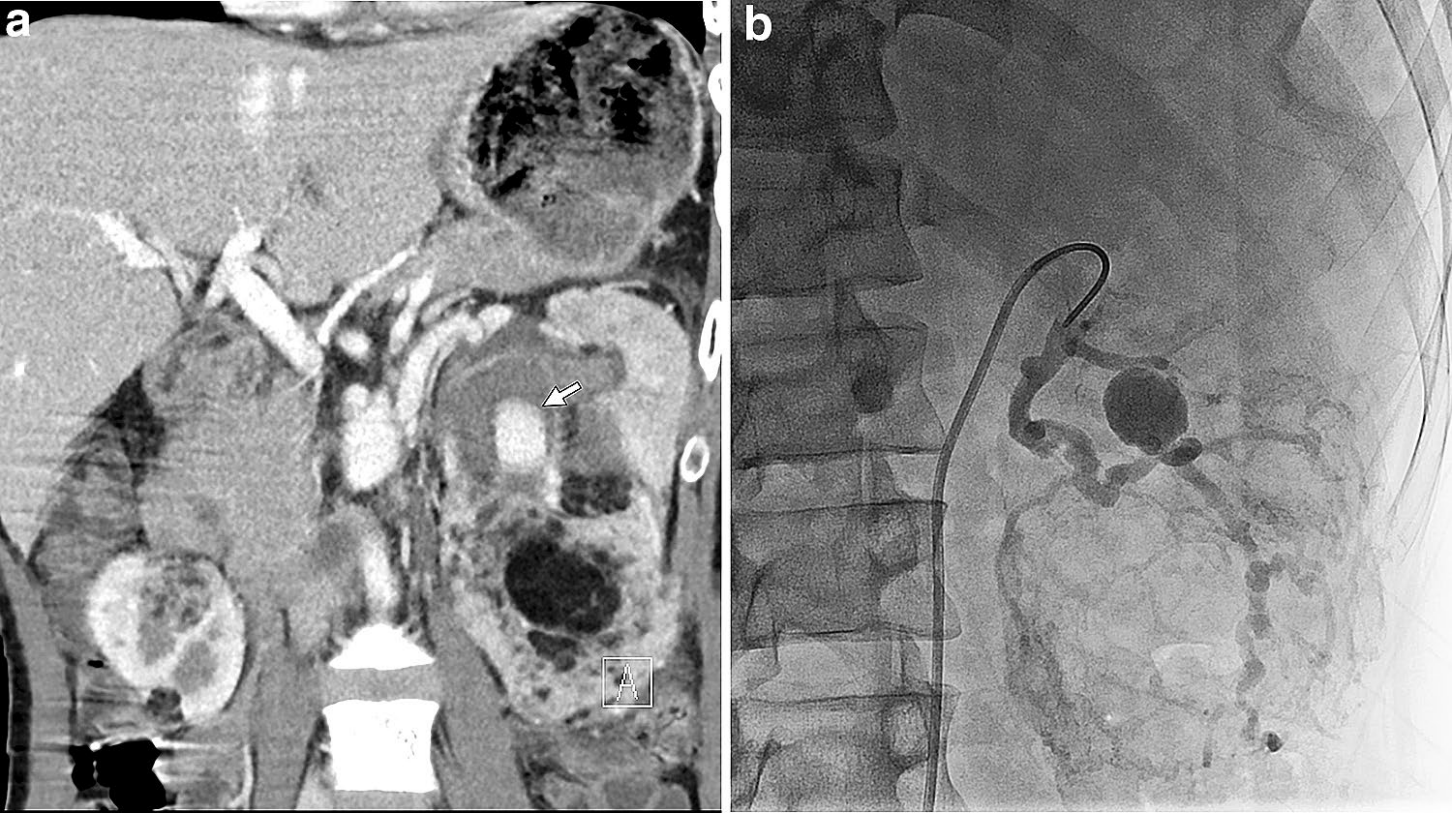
TAE for renal AML in TSC in a 29-year-old male with TSC and mental retardation. A multiplanar reconstruction coronal image of post-contrast abdominal computed tomography (a) shows multiple AMLs in both the kidneys and an aneurysm (arrow) within an AML in the left kidney. Note that a motion artifact is shown due to patient body motion caused by mental retardation. In this patient who could not hold his breath under sedation, we did not plan to acquire DSA but only acquired free-breath DA of the left renal artery with HF (b), where no respiration-related misregistration is shown. Background overlap is a little marked in this image acquired with the DDO set value of 60%, which is lower than the routine value (i.e., 70–90%)
